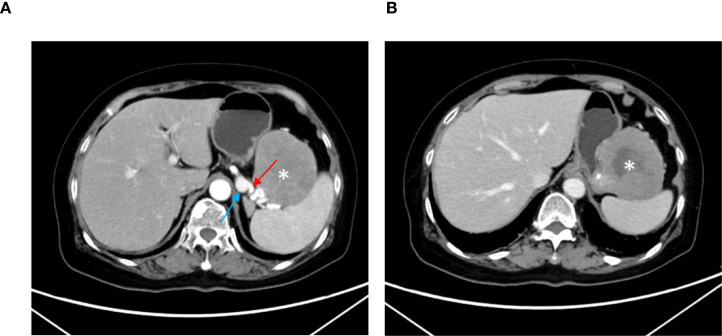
Contrast-enhanced abdominal CT scan of the gastric mass. (A) The exophytic mass at the fundus and the greater curvature (asterisk), with indistinct boundaries separating it from the splenic artery (red arrow) and splenic vein (blue arrow); (B) The mass contained sparse high-density foci and areas of liquefactive necrosis (asterisk) cm.
